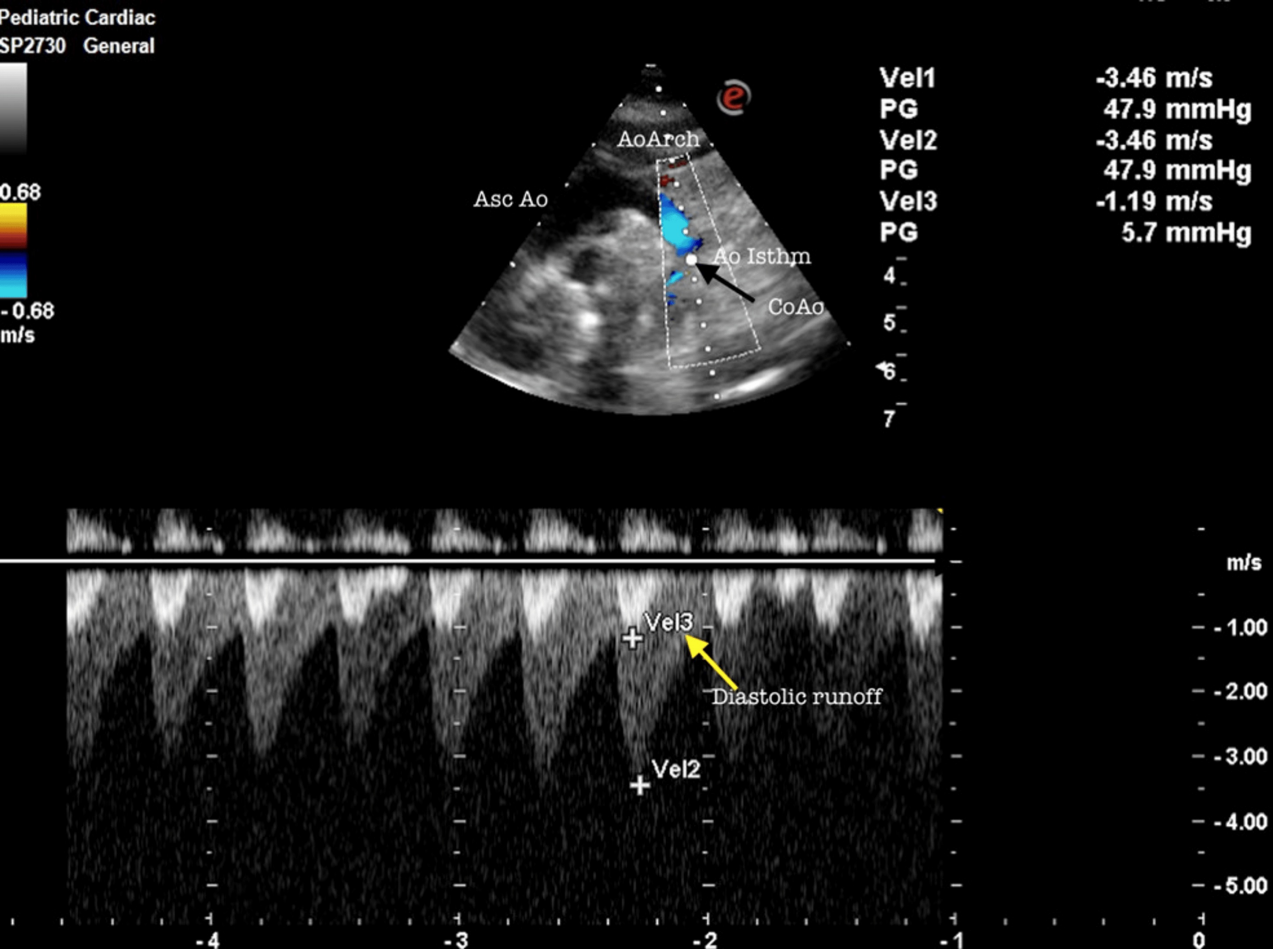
Coarctation of the aorta Suprasternal view in transthoracic echocardiography showing flow velocity acceleration with diastolic runoff (yellow arrows) in the distal 5th aortic arch. AscAo: Ascending aorta; AoArch, aortic arch; Ao isthm: Aortic isthmus; CoAo: Coarctation of the aorta
